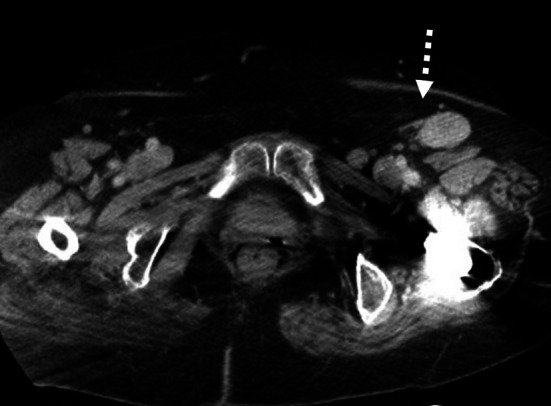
The increasing size and numbers of left inguinal lymph nodes were clearly presented in enhanced pelvic CT. The finding was first detected 46 months after the breast cancer operation
